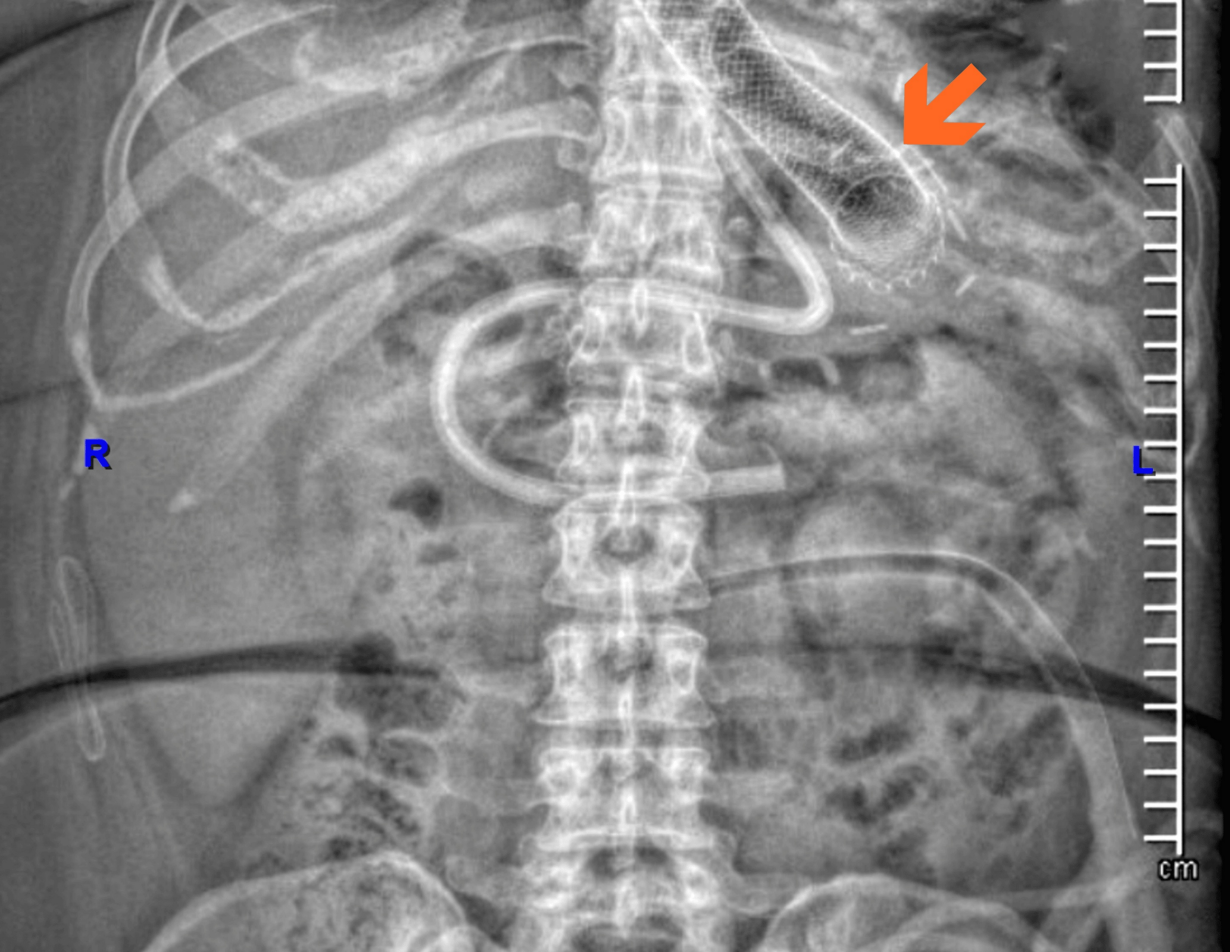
Plain Abdominal Radiograph After Placement of Covered Self-Expandable Esophagogastric Stent. Anteroposterior plain abdominal radiograph showing a covered self-expandable metallic stent in an appropriate intraluminal position at the esophagogastric junction. No signs of migration or pneumoperitoneum are observed. Intra-abdominal tubes and drains are also visible, consistent with ongoing postoperative management.
